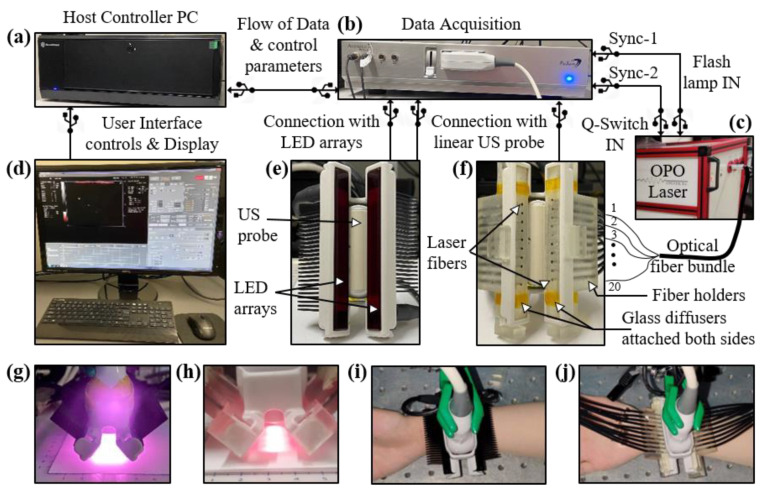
Description of the experimental setup designed for comparing light-emitting diode (LED)-based and high-power laser-based photoacoustic (PA) and ultrasound (US) imaging. The setup consists of the following key components: Commercial B-mode LED-PAUS system (AcousticX, Cyberdyne Inc., Ibaraki, Japan) with (a) a host controller PC and (b) data acquisition hardware. (c) A portable high-power laser (Phocus Mobile, Opotek Inc., Carlsbad, CA, USA) with its output coupled to the input end of an optical fiber bundle. The fiber bundle is split into twenty smaller fibers at the distal end. (d) Computer display: displays B-mode US (grayscale), PA (red scale), and coregistered US + PA (overlaid red PA on gray US). The interface also enables switching between LED and laser operation. (e) Arrangement of two 850 nm LED arrays around the US probe. (f) Arrangement of twenty laser fibers inserted into the two fiber holders around the US probe. Two glass diffusers attached at the fiber output ends to provide uniform laser illumination on the tissue surface. Optical illumination profile achieved with (g) two LED array sources and (h) laser source. (i,j) Pictures of a human wrist under imaging with the LED and laser arrangements, respectively.
